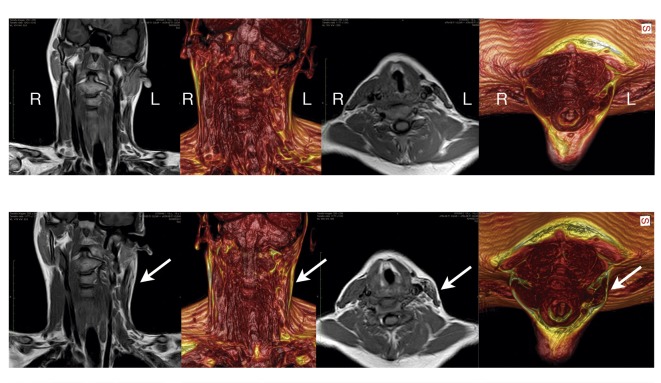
Preoperative and postoperative MRI of Case 1 Upper row: preoperative MRI images. Lower row: postoperative MRI images at six months (arrows point out treated muscle).
